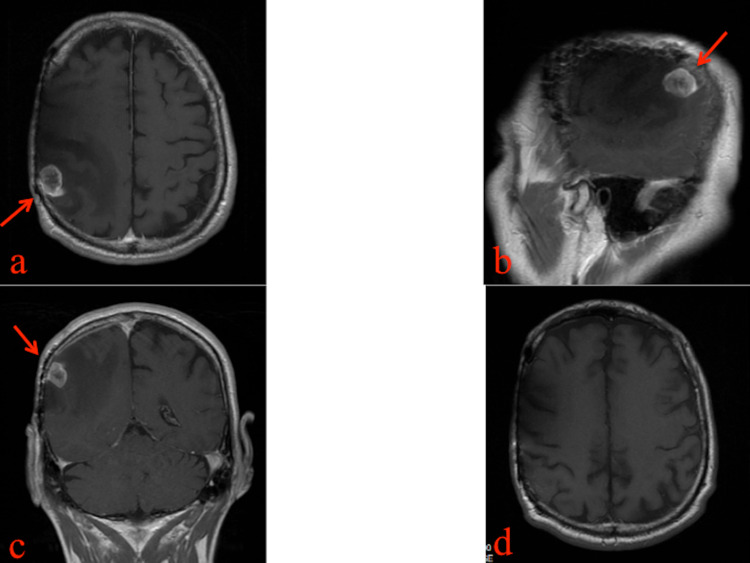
Pre and postoperative brain MRI Post-contrast axial T1 (a), post-contrast sagittal T1 (b), post-contrast coronal T1 MRI sequences (December 18, 2019) showed a metastatic, cortico-subcortical, right temporoparietal lesion with profuse edema. Postoperative post-contrast axial T1 MRI sequence (d).
